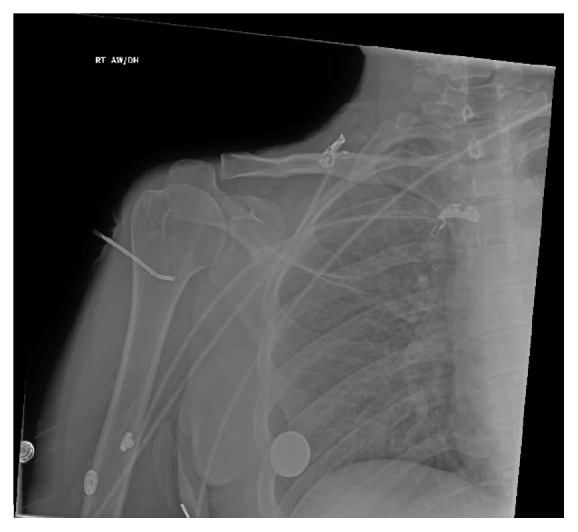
Radiograph of the right shoulder with a bent intraosseous needle in the neck of the humerus.
